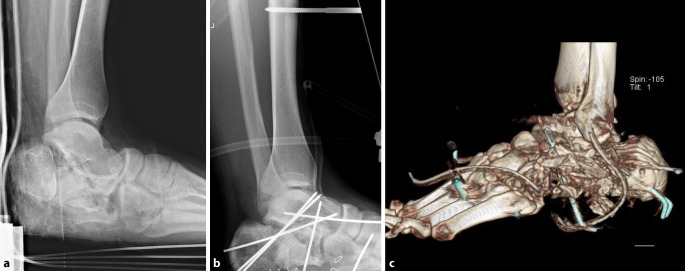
Radiologisch serielle Frakturen des linken Rück- und Mittfußes. a Kalkaneusluxationsfraktur (Typ 5, 3.° offen) mit Kuboidimpressionsfraktur und Lisfranc-Brüchen. Aufnahme am Tag des Unfalls. b Notversorgung mit Débridement, Adaptations-K-Draht-Osteosynthese, tibiotarsaler Transfixation und Kunsthautdeckung. c CT-Kontrolle. (2 Tage nach Unfall)
